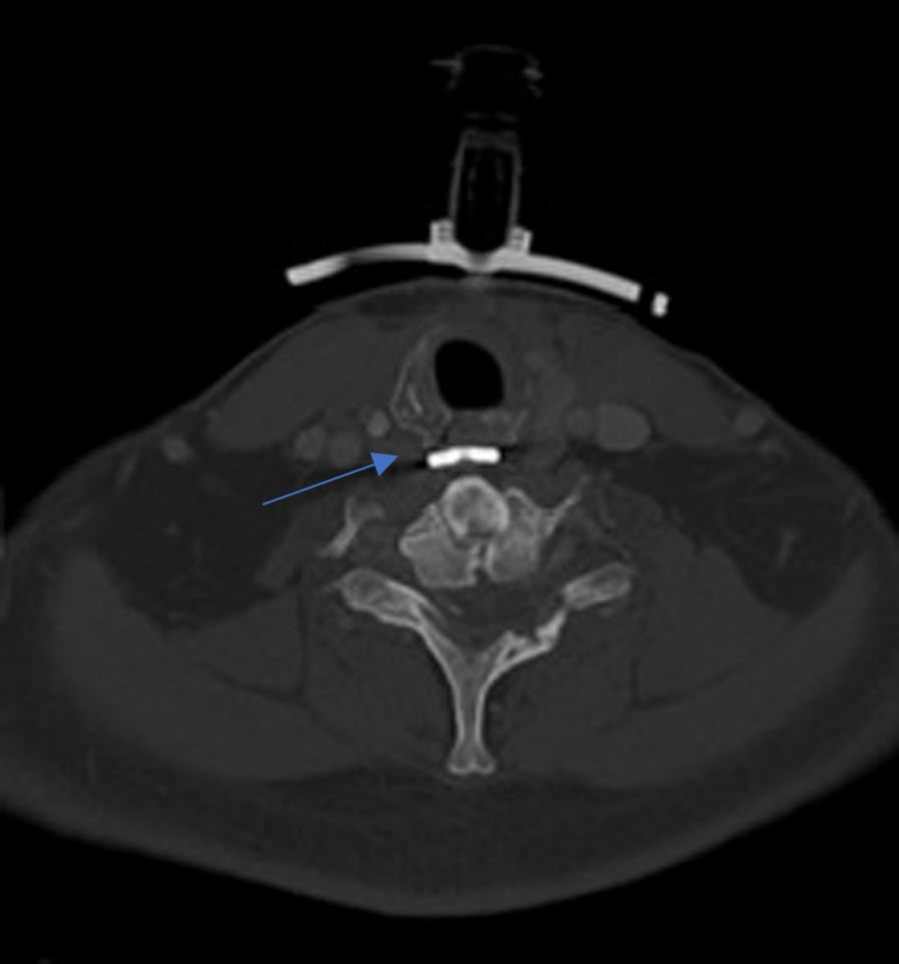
Cervical CT. Necrosis of the lamina of the cricoid cartilage, next to the osteosynthesis material (blue arrow)
